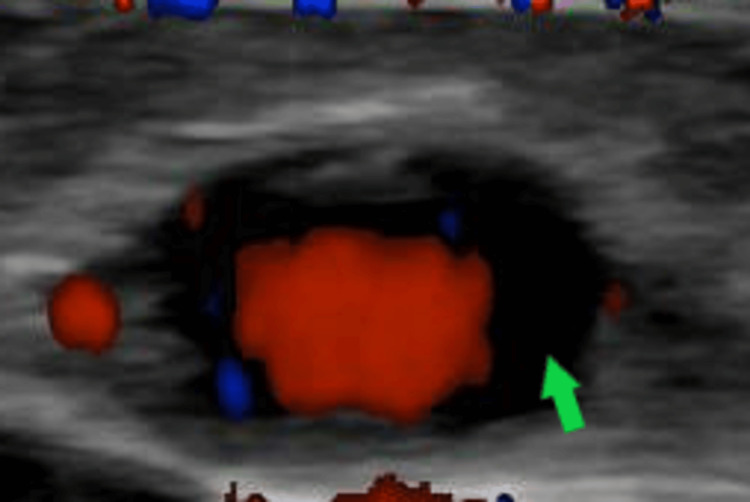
Colour Doppler ultrasound of this patient showing a transverse view of the frontal branch of the left temporal artery which demonstrates a classic "halo sign" representing the inflamed and swollen arterial walls that is a hallmark of GCA GCA - giant cell arteritis
